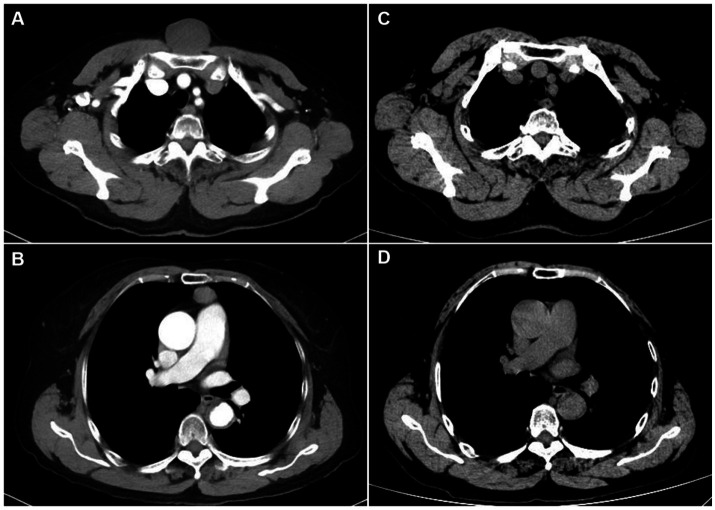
Preoperative and follow-up chest computed tomography (CT) images. (A) On 26 March 2023, chest CT demonstrates a well-defined, round subcutaneous lesion (approximately 44 mm × 43 mm) anterior and superior to the manubrium. (B) On 26 March 2023, chest CT reveals a well-defined mass (approximately 22 mm × 16 mm) in the anterior mediastinum. (C) On 11 October 2024, follow-up chest CT shows no evidence of tumour recurrence at the previous subcutaneous site. (D) On 11 October 2024, follow-up chest CT shows no evidence of tumour recurrence at the previous anterior mediastinal site.
